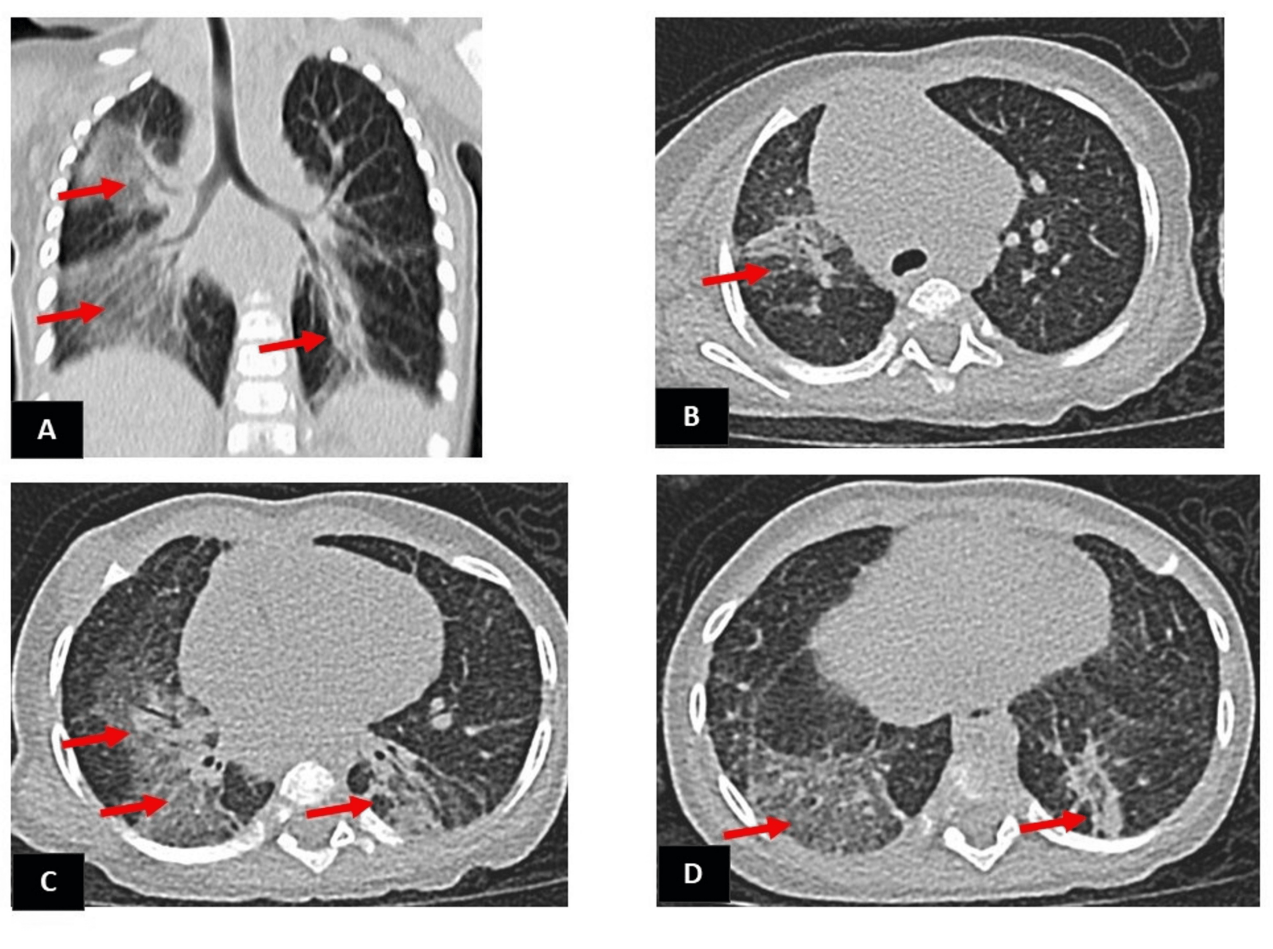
High-resolution CT chest performed at six months of age (A) Coronal oblique image (red arrows) shows bilateral patchy ground-glass densities containing plate atelectasis involving the upper, middle, and lower lobes on the right side in addition to the left lower lobe. (B) The image demonstrates an ill-defined ground-glass opacity in the anterior segment of the right upper lobe (red arrow). (C) Patchy ground-glass opacities are seen in the medial segment of the right middle lobe and the superior segments of both lower lobes (red arrows). (D) The image shows an ill-defined ground-glass opacity in the superior segment of the right lower lobe and plate atelectasis in the superior segment of the left lower lobe (red arrows).
